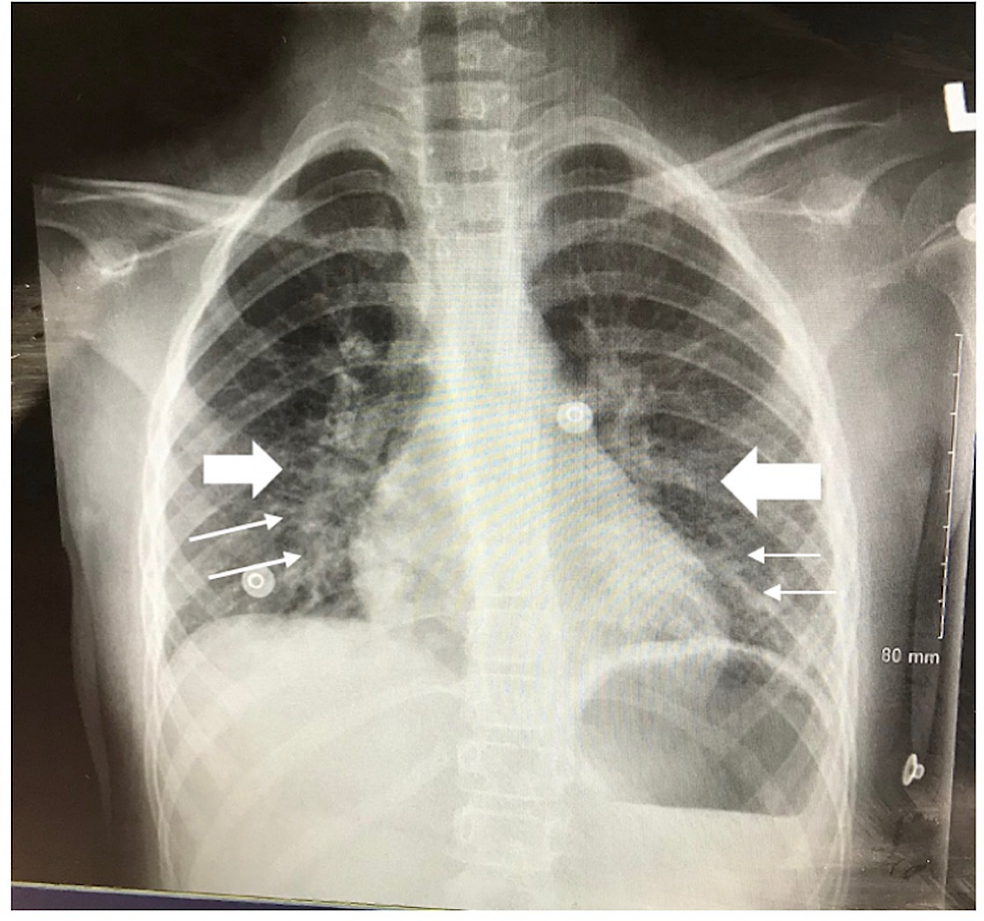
Anterior Posterior (AP) chest x-ray showing bilateral basal infiltrates concerning atelectasis.
